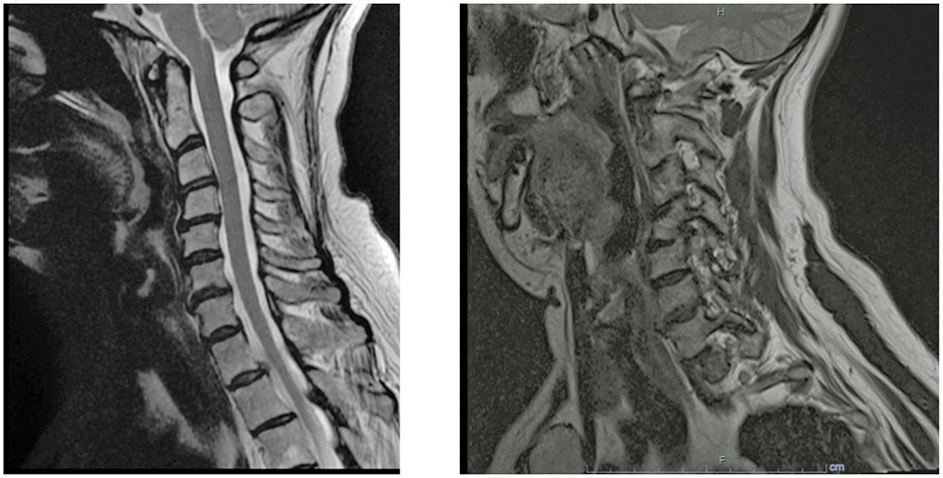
MRI impression: Bilateral foraminal stenosis at C4 and C5, related to uncovertebral arthropathy, with exiting C5 nerve root impingement. At C5–C6, there is significant right foraminal stenosis with exiting C6 nerve root impingement. Photo courtesy of Joseph A. Marchione, MD, Neuroradiologist at Garnet Health Medical Center.
